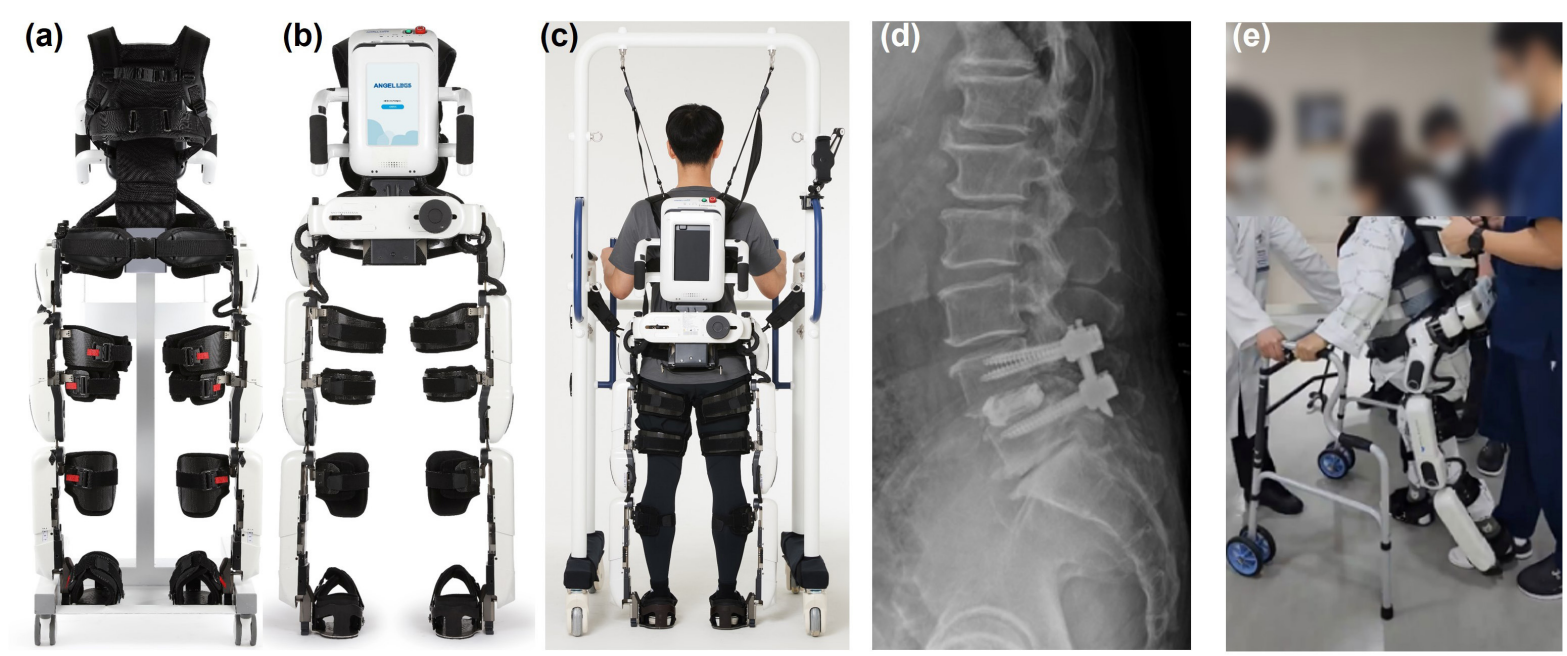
Exoskeletal wearable robot and patient who underwent RAGT. (a) Anterior and (b) posterior views of the robot. (c) Posterior view of a patient wearing the robot and using a harness. (d) Lateral lumbar radiograph and (e) image shows a patient undergoing RAGT while wearing a spinal orthosis.
